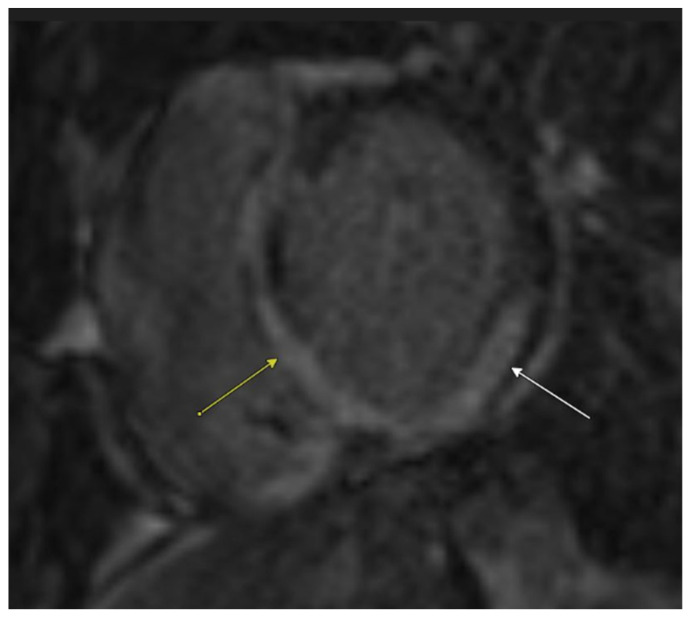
Cardiac MRI with late post-gadolinium-enhanced inversion recovery imaging in a short axis view at the base demonstrates extensive abnormal left ventricular myocardial late enhancement in a mid-wall and epicardial predominant distribution, particularly involving the basal inferior wall, septum (yellow arrow), and inferolateral wall (white arrow).
